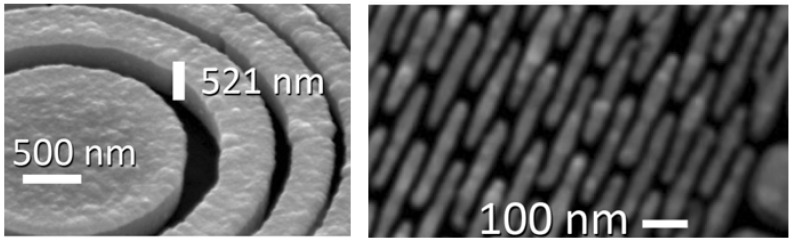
SEM images (from Reference 7) of a recent Au hard-X-ray FZP.
